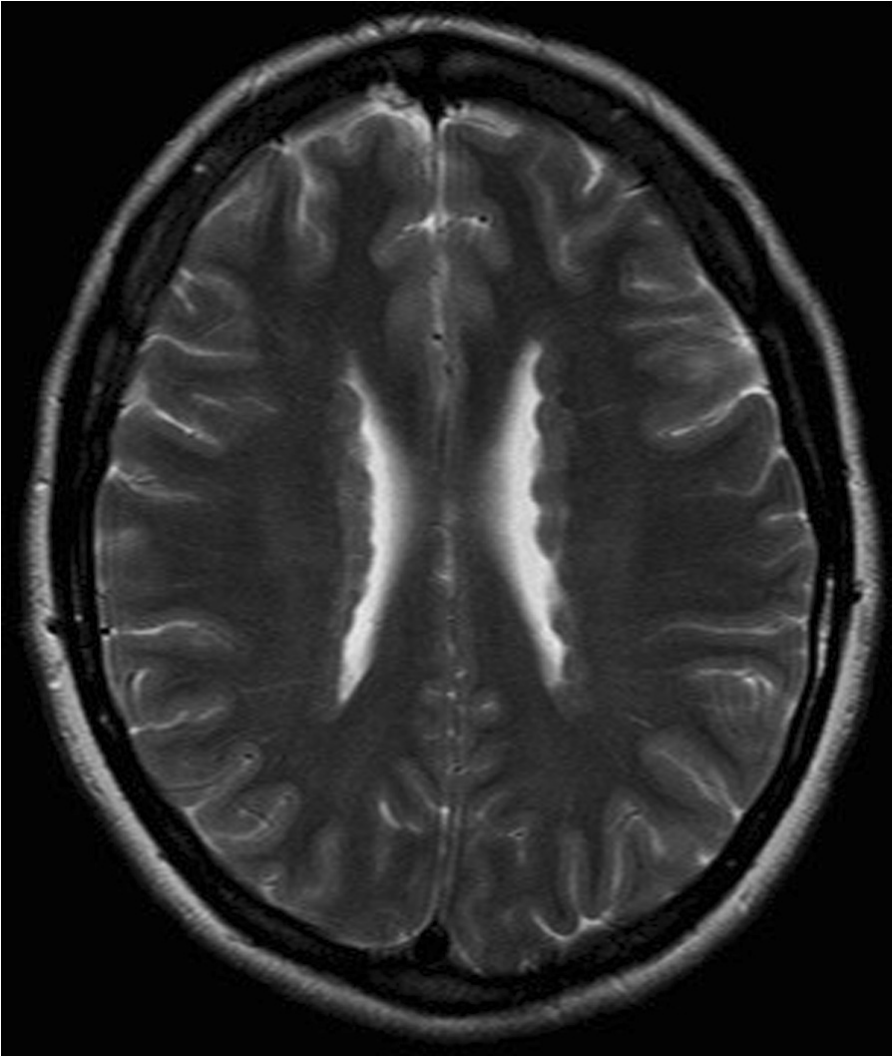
Brain MRI of the sister showing PNH.
